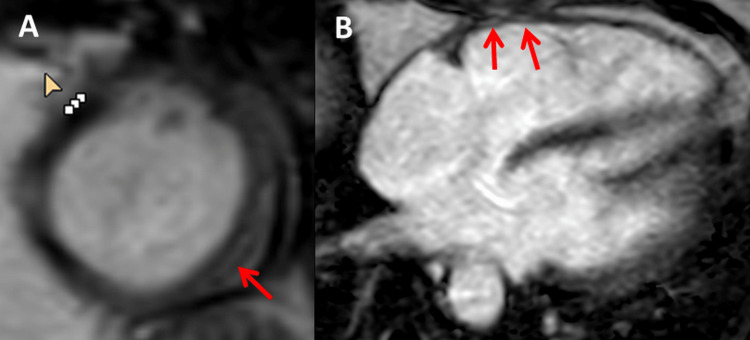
Cardiac MRI at age 49 years showing subepicardial LGE 4-12 minutes after intravenous application of gadolinium in the lateral and posterior wall of the left ventricular myocardium on 3D-T1-TFE sequences (panel A) and on T1w-PSIR sequences (panel B) LGE: late gadolinium enhancement; 3D: three dimensional; TFE: turbo field echo; PSIR: phase-sensitive inversion recovery
